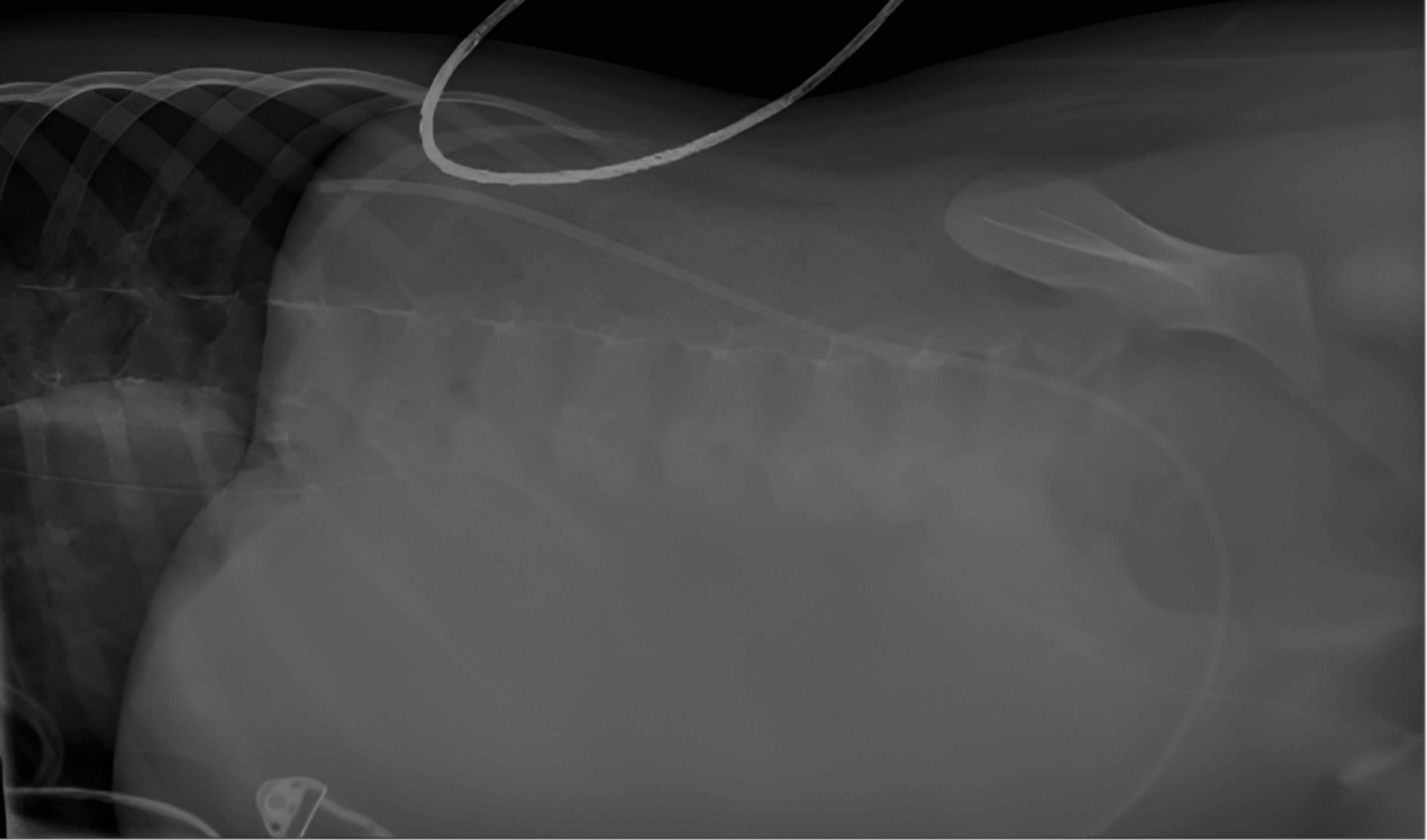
Lateral supine plain abdominal radiography. Abdominal air leakage is absent
